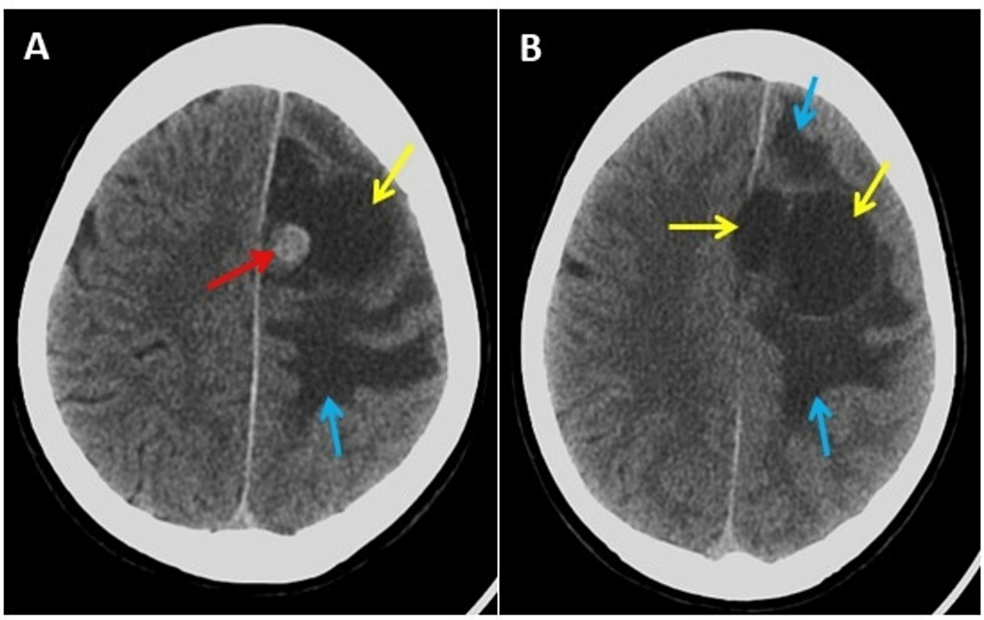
Brain computed tomography scan without contrast. Axial (A) and (B) lower level showing a left frontal multicystic lesion (yellow arrows) with a small rounded hyperdense nodule (red arrow) and surrounded with massive edema (blue arrows).
